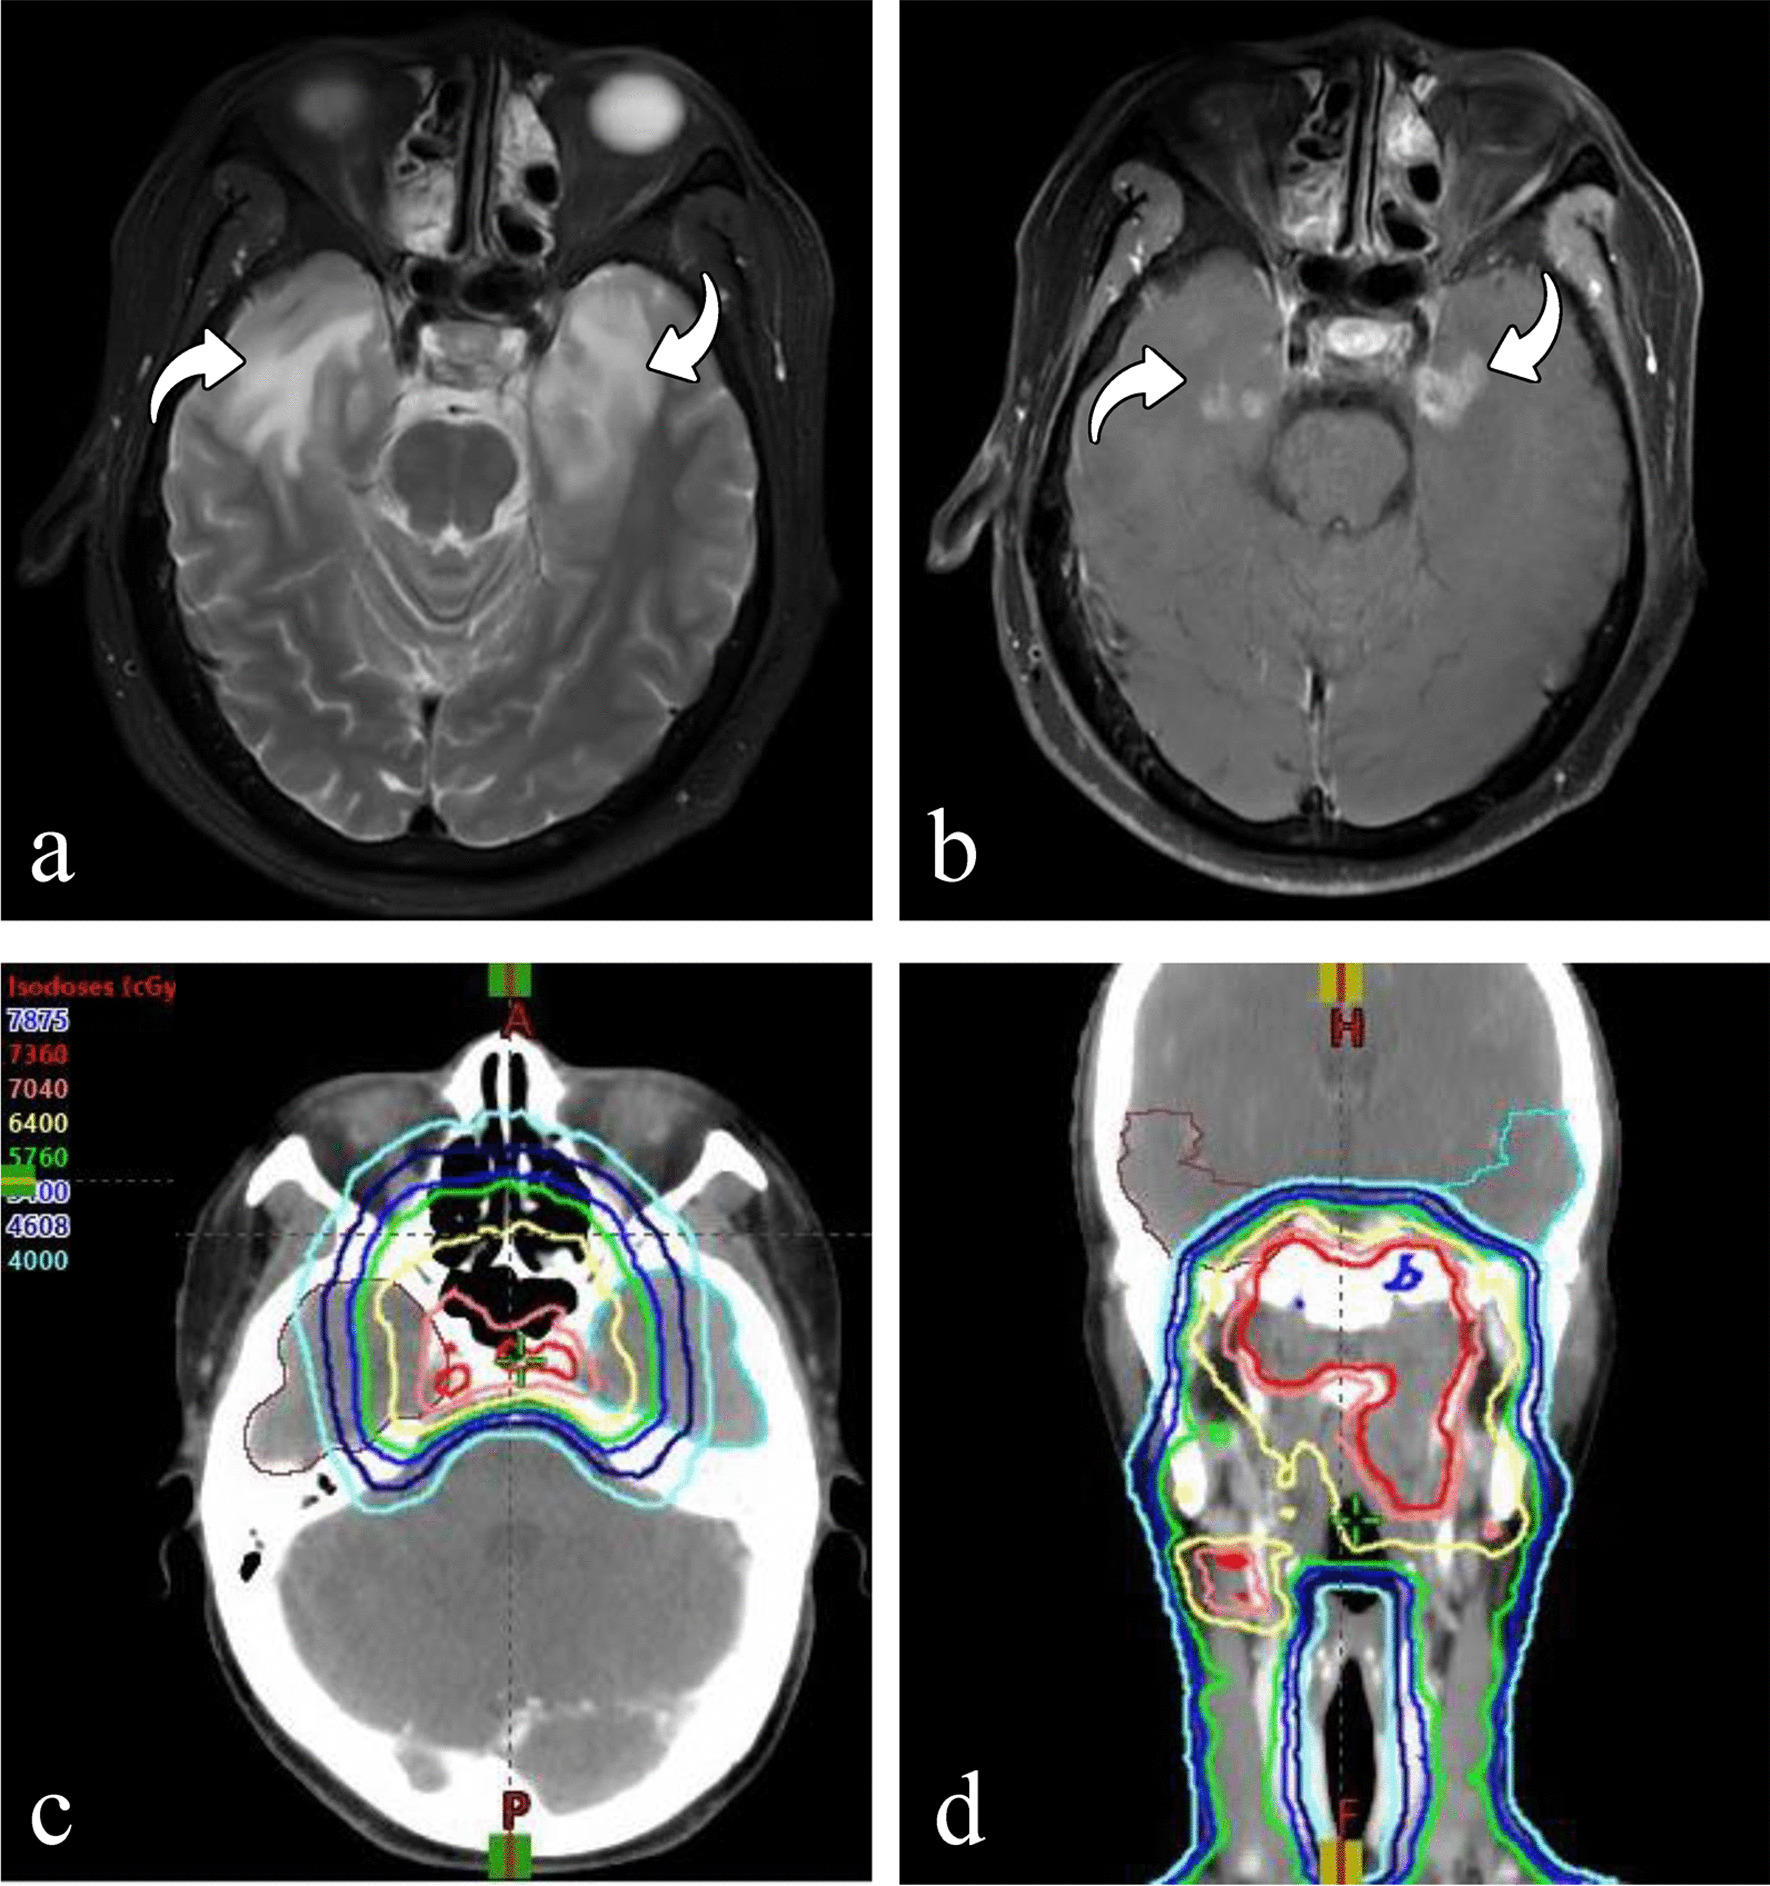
A representative example of white matter lesion in bilateral temporal lobes and dose distribution of the treatment target area treated with three‑dimensional radiotherapy. a High intensity in the white matter of bilateral temporal lobes on axial T2-weighted image (curved arrow), b contrast-enhanced lesions on axial postcontrast T1-weighted MR image (curved arrow). Dose line distribution is shown in c (axial) and d (coronal), respectively
